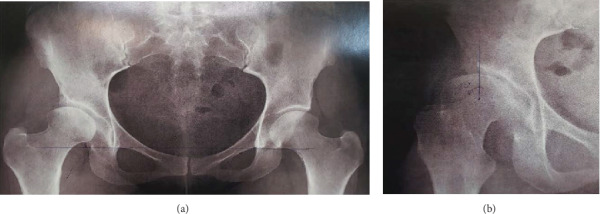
Radiographs of the pelvis (AP in (a) and Lequesne view in (b)) showing signs of hip dysplasia.
